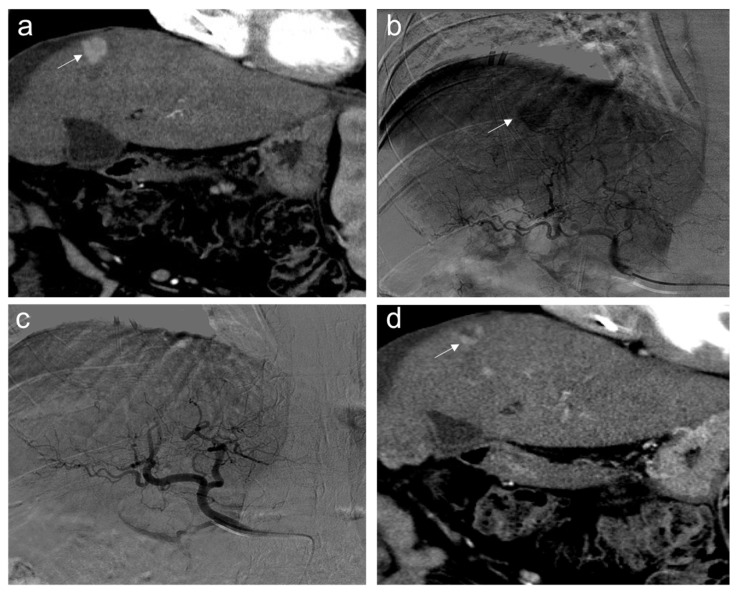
Partial response. Sixty-five-year-old man, affected with HCC, expresses low miR-4492 levels at the time of diagnosis and undergoes TACE procedure: (a) pre-treatment arterial contrast-enhanced CT shows a 22 mm nodule (arrow) in segment 8 with arterial hyper-enhancement; (b) selective angiography confirms a hypervascular mass in segment VIII (arrow); (c) after TACE, the lesion was no longer visible; and (d) one month after the procedure, the presence of nodular hyper-enhancement in the arterial phase (arrow) demonstrates the residual viable tumor consistent with a partial response to treatment.
